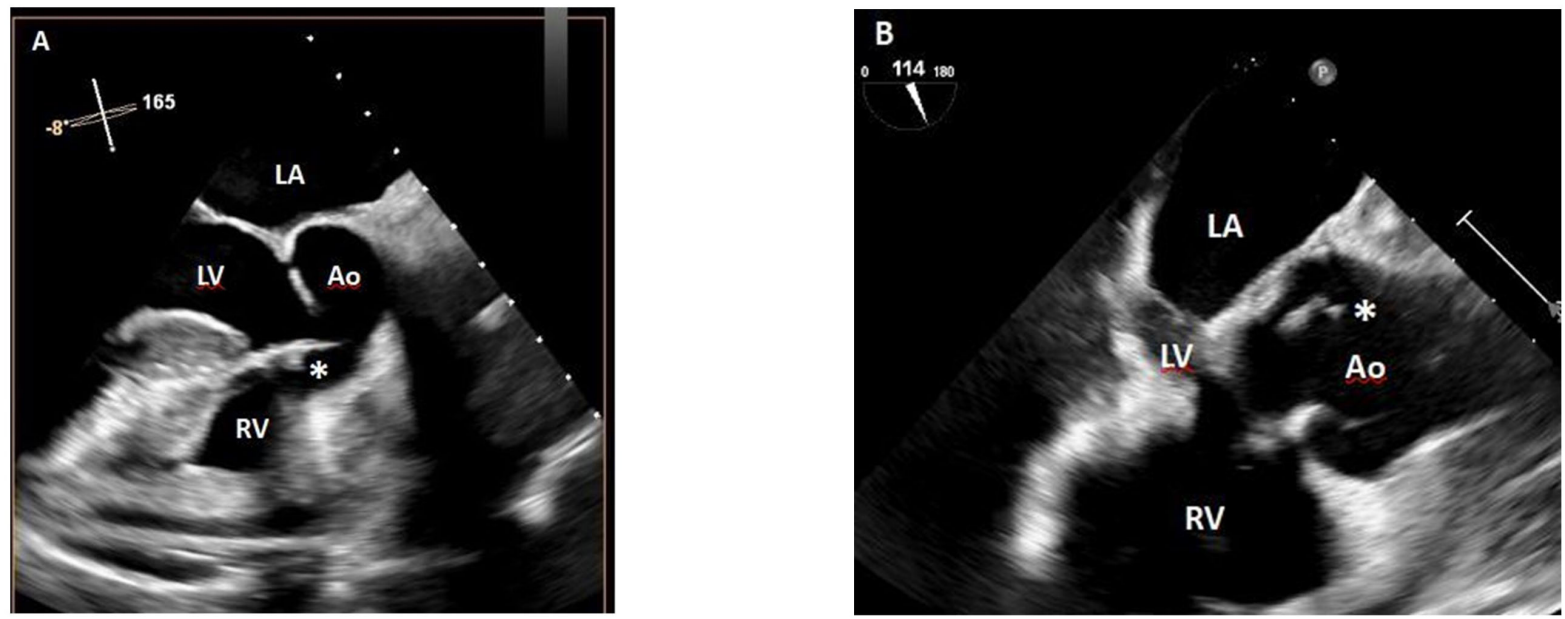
Transesophageal echocardiography (TEE) (A) reveals small vegetations (0.19-0.46 cm) on the aortic valve, and follow-up TEE (B) shows partially resolved oscillating vegetations (0.17-0.29 cm).
